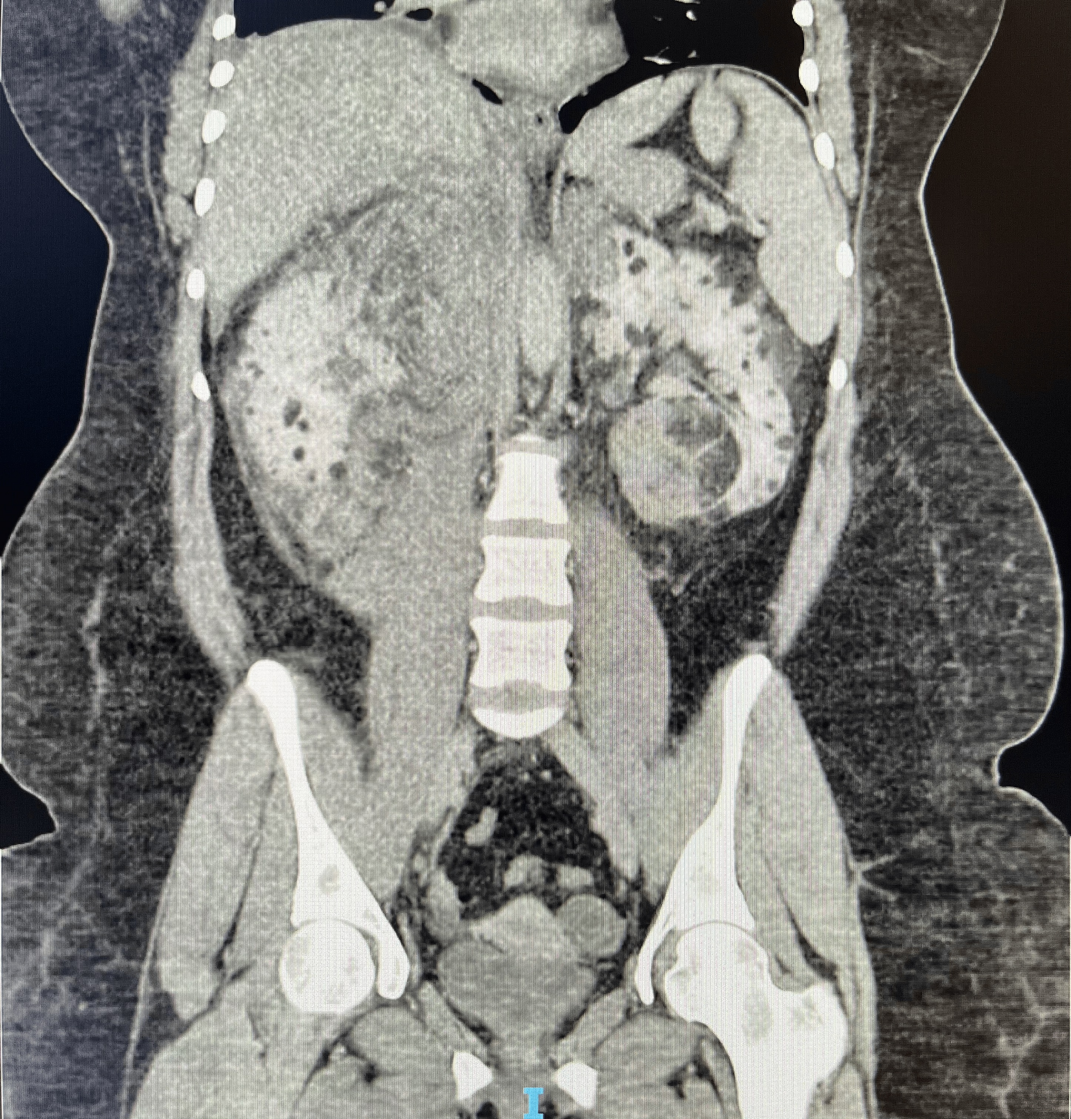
Index CT kidney triple phase: arterial phase with coronal views of the abdomen The figure highlights the extent of intrarenal AMLs. There are innumerable bilateral renal angiomyolipomas with multiple intra-tumoral haemorrhages, as demonstrated in the inferior pole of the left kidney. AML: angiomyolipoma
